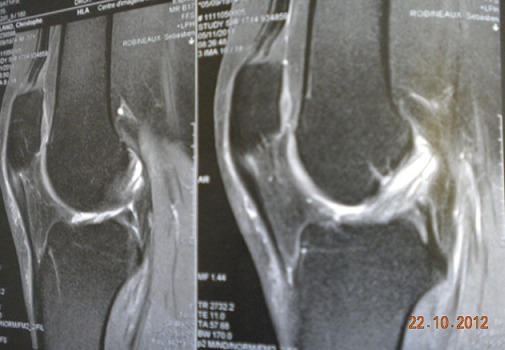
Sagittal T2-weighted image showing increased intraligamentous signal intensity of the ACL.
